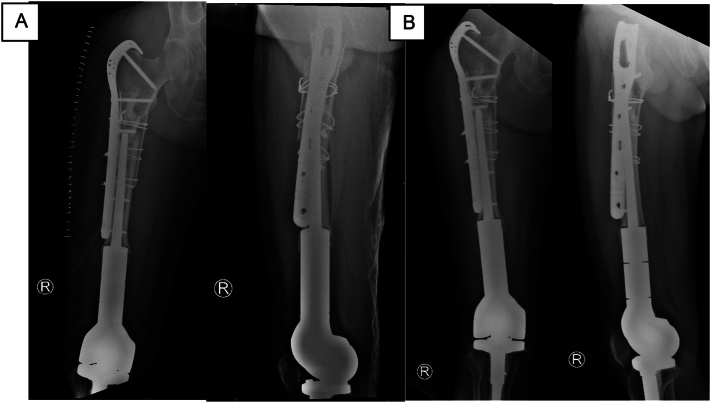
Post-operative radiograph. (A) 1-day post operative, (B) 6-weeks post operative.
